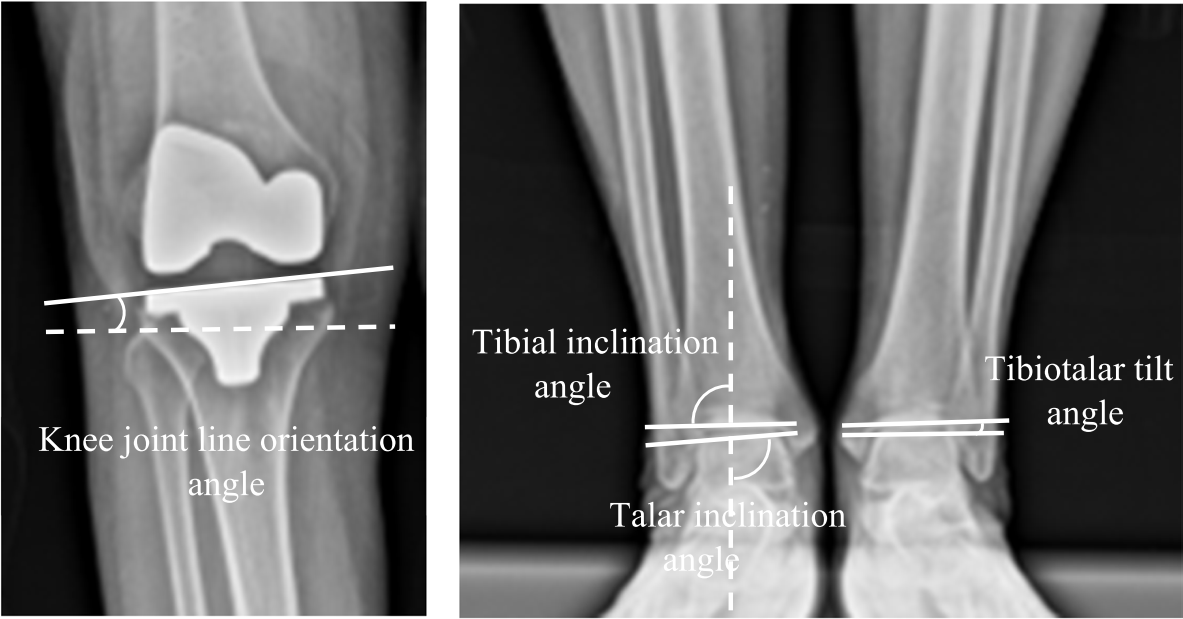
Radiographic measurements: (a) knee joint line orientation (KJLO) angle, which had a positive value when the joint line was slanted down to the lateral side; (b) Tibial inclination, talar inclination, and tibiotalar tilt angle
